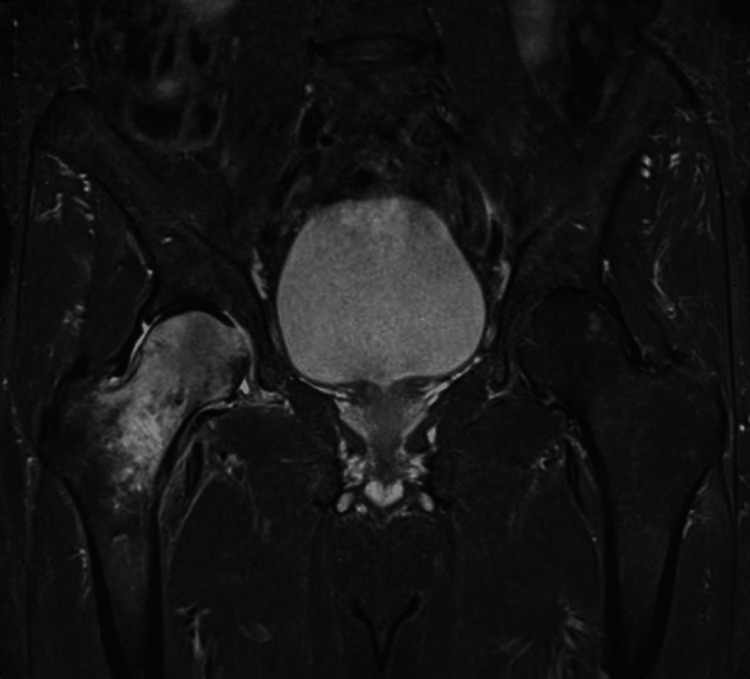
Hip osteoporosis image by magnetic resonance imaging
